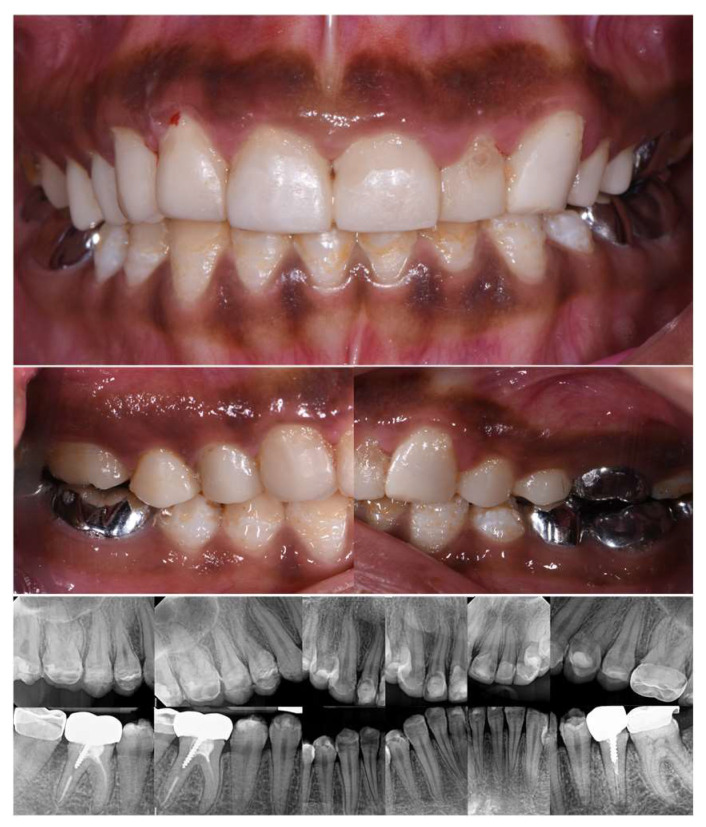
Intraoral views and radiographs at 1.5-year follow-up.
